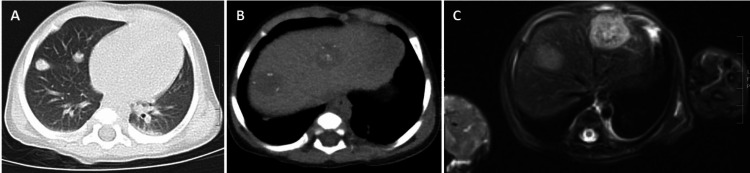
Multiple lesions are identified in the lung CT (A), liver CT (B), and MRI (C) CT: computed tomography; MRI: magnetic resonance imaging
